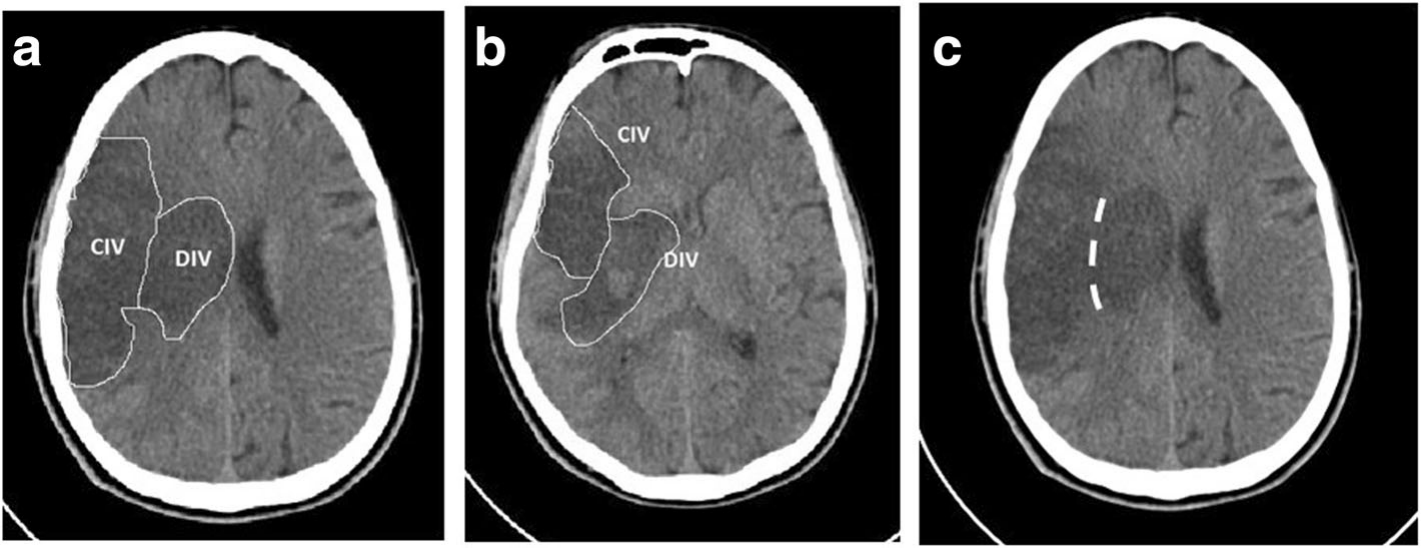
Representative CT images of the segmentation between the cortical and deep infarct areas. a + b demonstration of CIV and DIV, c demonstration of the natural separating line of the corona radiate. *CIV* cortical infarct volume in ml, *DIV* deep infarct volume in ml
